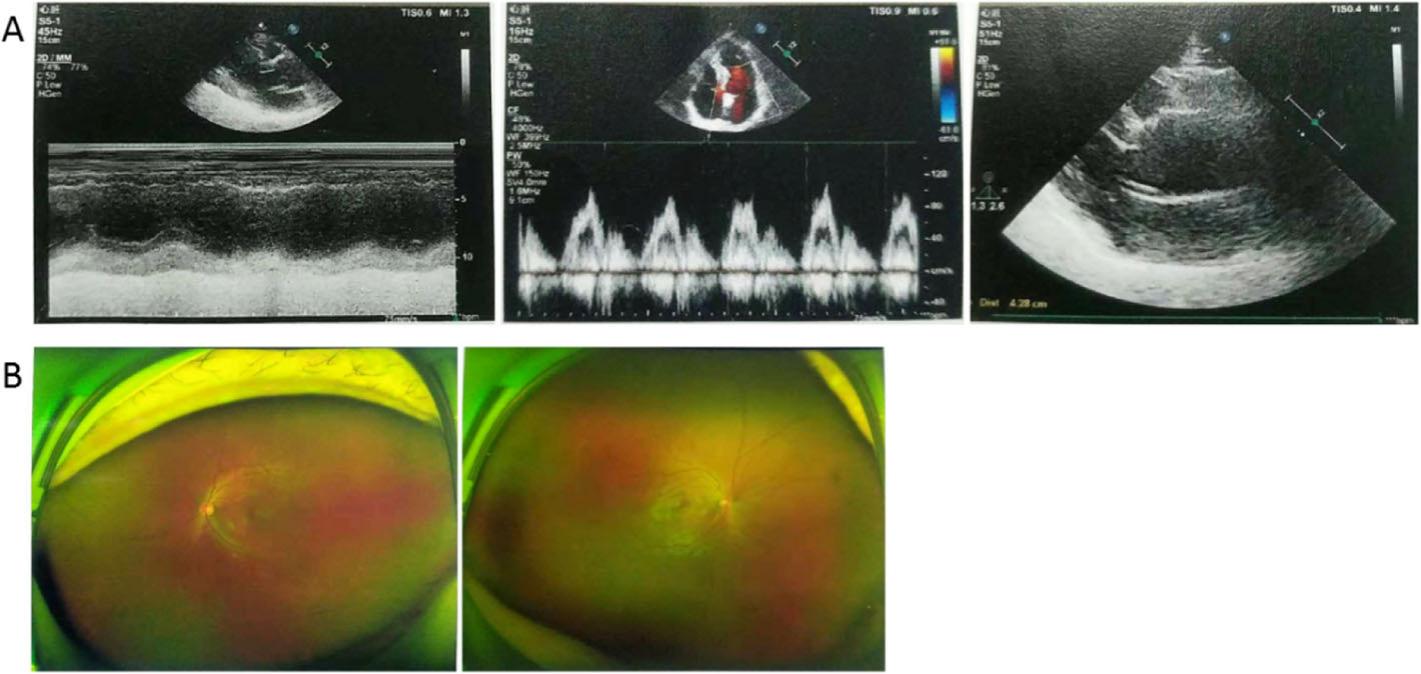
Clinical diagnosis of the proband. 2A: Ultrasonic cardiogram; 2B: Ocular ultrasound. Echocardiography showed dilatation of ascending aorta and main pulmonary artery, mitral regurgitation (mild), tricuspid regurgitation (mild), and abnormal left ventricular filling (a). Ocular ultrasound showed vitreous opacity (b)
